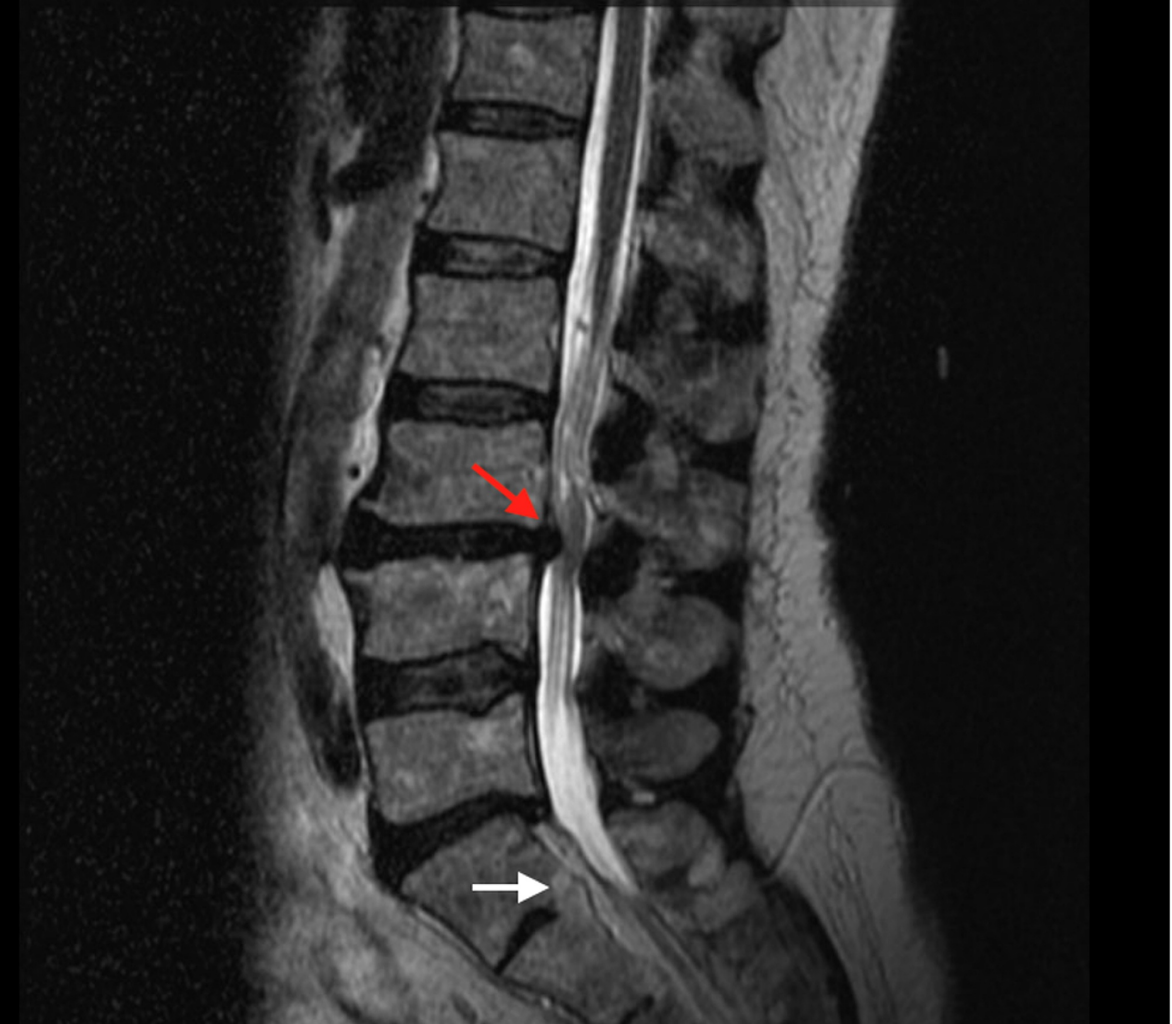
MRI of Lumbar Spine. A mid-sagittal T2-weighted MRI of the lumbar spine with degenerative changes including a disc protrusion at L3-4 (red arrow) and partial auto-fusion of L5-S1 (white arrow), though these may represent surgical changes.
